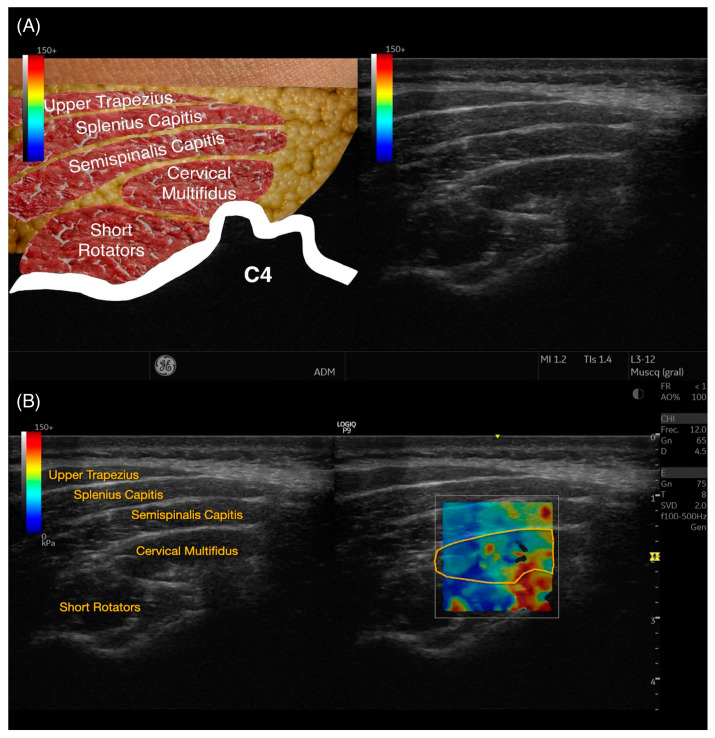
Raw ultrasound imaging acquired at C4–C5 level (A), contouring the targeted structure (cervical multifidus) and references used (articular pillar of C4–C5), and shear wave elastography imaging (B).
